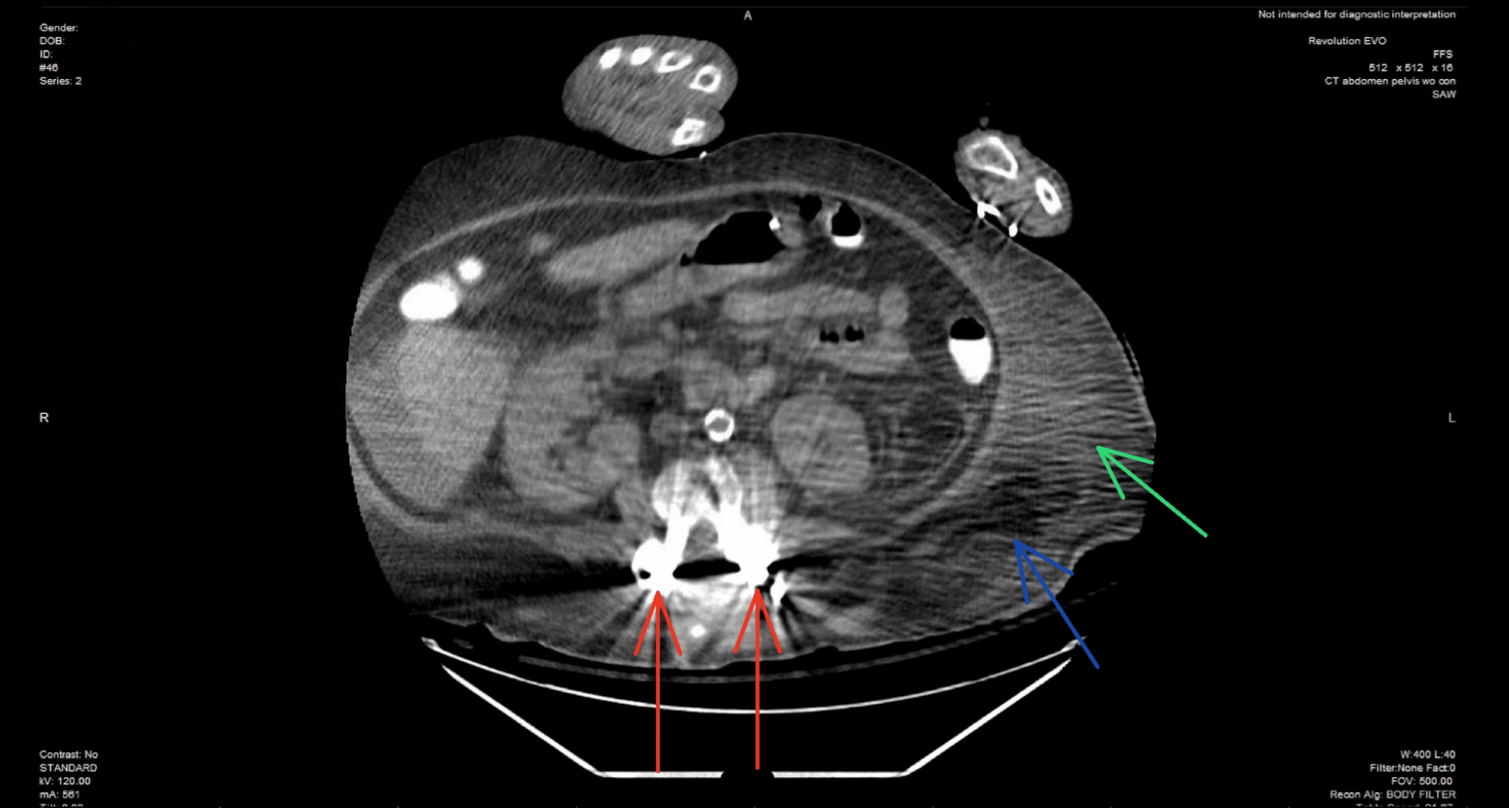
Axial view at the level of the spine, CT scan of the abdomen/pelvis shows spinal hardware implants (red arrows), subcutaneous emphysema (blue arrow), and subcutaneous fat standing (green arrow) CT: computed tomography
